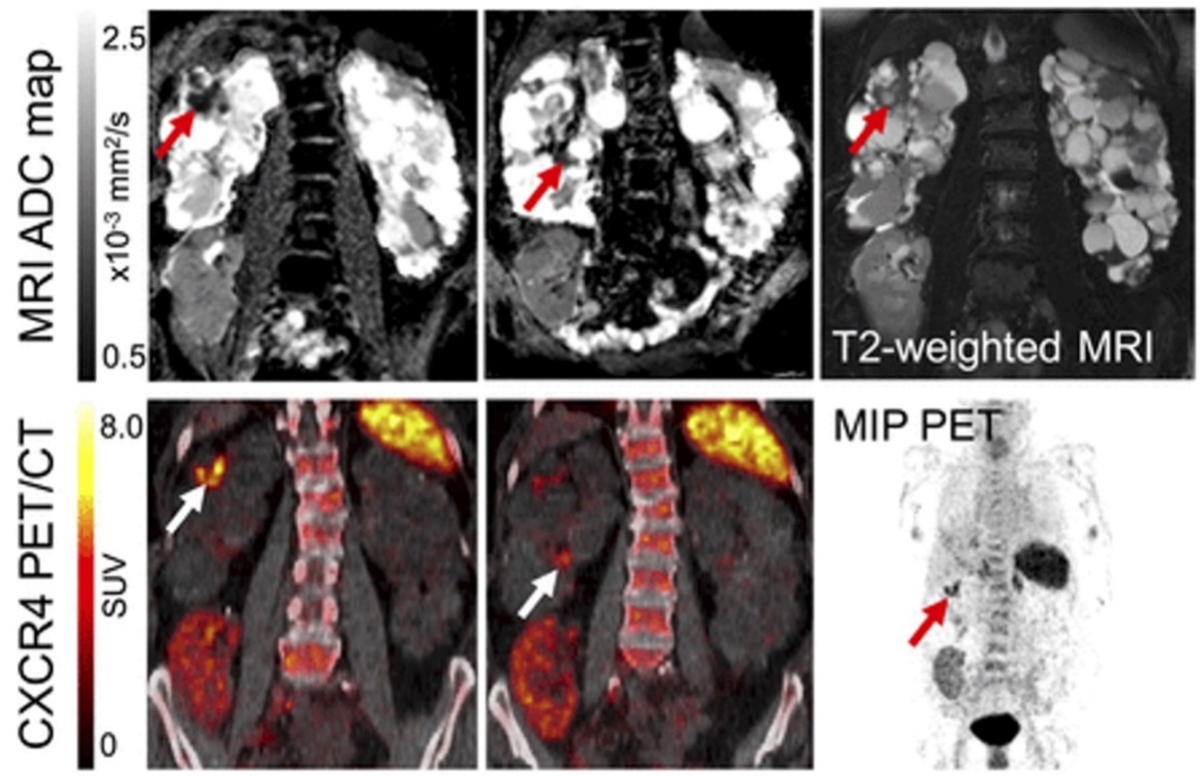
PET and MR of acute renal cyst infection. MR (top) and PET/CT images (bottom) of patient with kidney transplantation due to autosomal-dominant polycystic kidney disease and suspected complicated urinay tract infection. Imaging revealed upregulated CXCR4 expression in 2 cysts (white arrow) showing renal cyst infection. Corresponding MRI confirms a cyst with thick wall (T2-weighted MRI, red arrow) and apparent diffusion coefficient (ADC) reduction in both areas, consistent with PET findings. Allograft in right lower abdomen, however, shows no signs of infection with low homogeneous ^68^Ga-Pentixafor signal. MRI shows linear ADC reduction and volume loss, consistent with scarring at upper pole. MIP = maximum-intensity projection. Modified from Derlin et al 16, copyright 2017 by the Society of Nuclear Medicine and Molecular Imaging, Inc.
